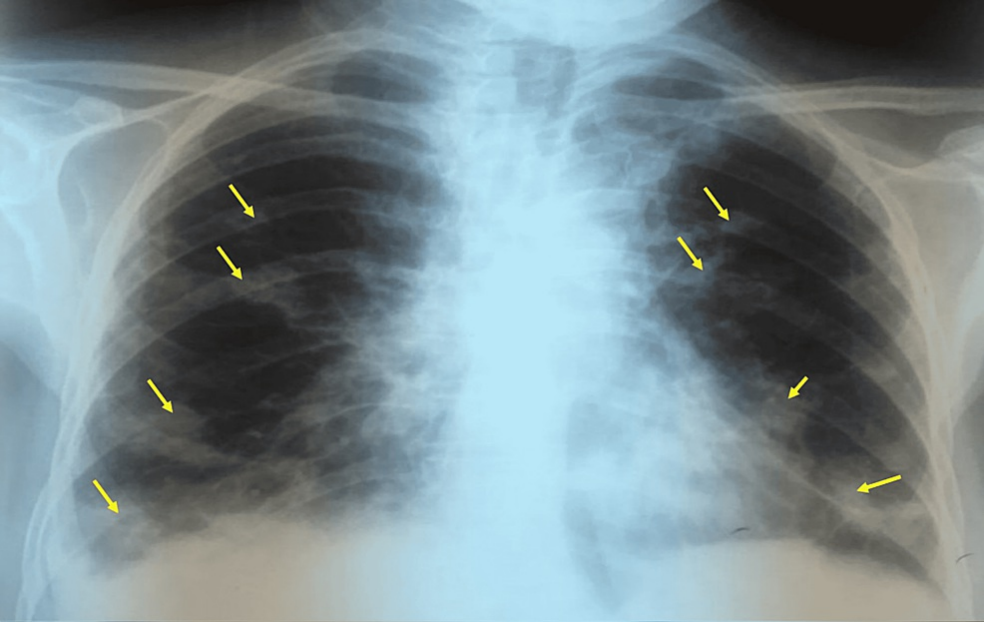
Anteroposterior chest x-ray (CXR) evaluating the cause for chronic productive cough showing multiple and disseminated rounded macronodular opacities of different sizes
